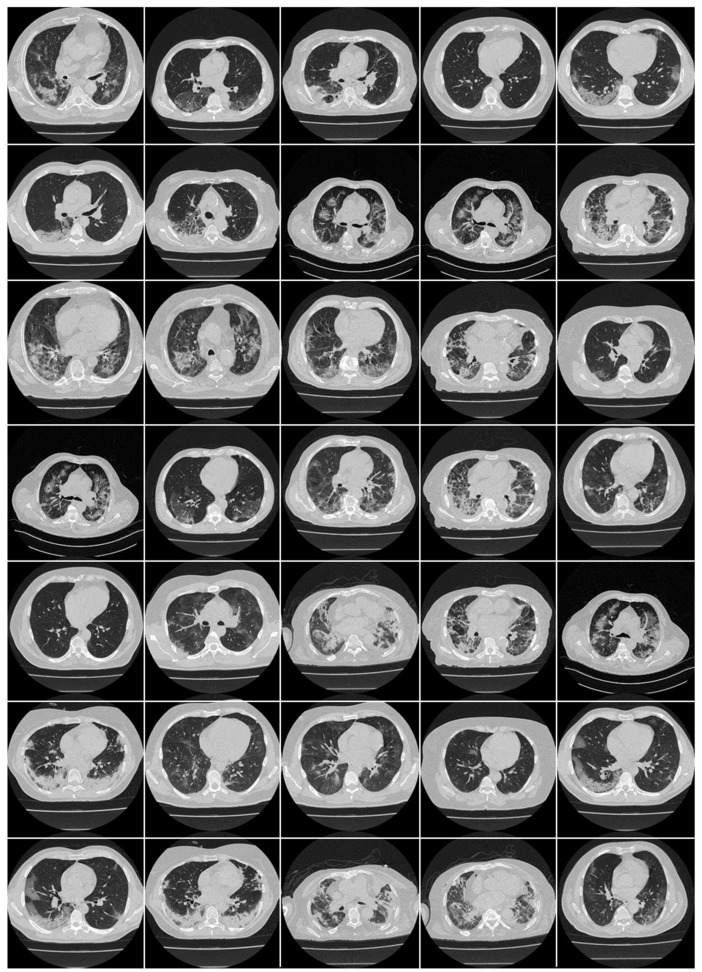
Raw CT slice of COVID-19 patients taken from Croatian data set.
